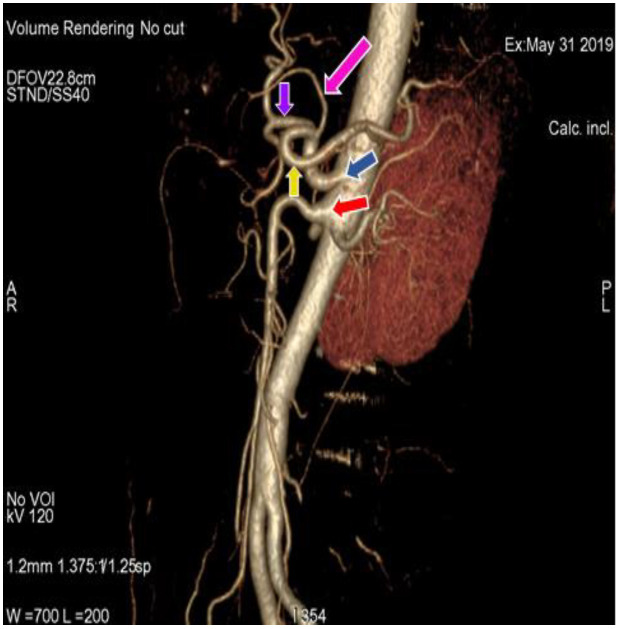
3D-Volume render (3D-VR) image of (64 row scanner CT angiography) abdominal region showing superior mesenteric artery (red arrow) and division of regular celiac trunk (blue arrow) into left gastric artery (pink arrow), splenic artery (yellow arrow) and common hepatic artery (purple arrow).
